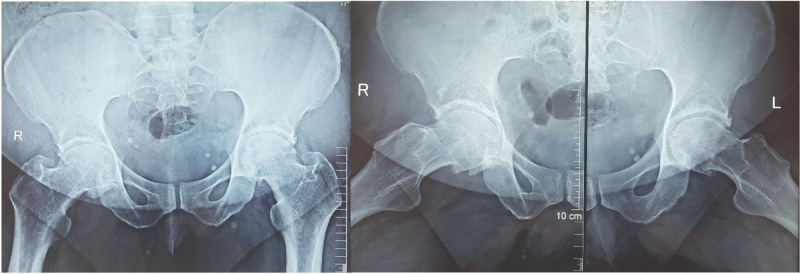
Anteroposterior radiographs of the pelvis and anteroposterior and lateral radiographs of bilateral hips at baseline showing severe bilateral hip osteoarthritis.
